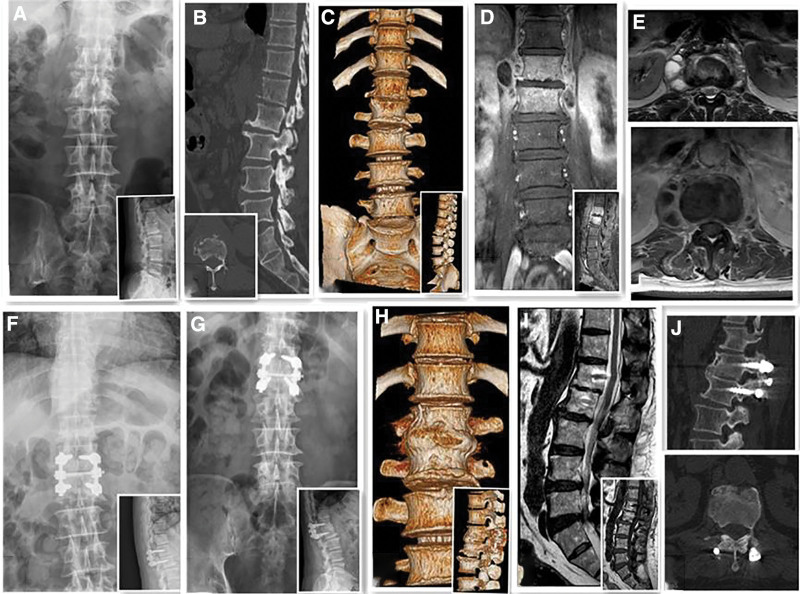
A 64-yr-old man, was admitted to the hospital mainly because of “low back pain with decreased muscle strength in both lower limbs” for 2 mo. Preoperative diagnosis revealed L1–2 brucellosis spondylitis, thus he was subjected to L1–2 simple bone graft internal fixation. (A) Preoperative X-ray films; (B and C) preoperative CT plain scan + reconstruction; (D and E) preoperative MRI; (F) X-ray films taken 3 mo after surgery; (G) lateral and frontal radiographs at last follow-up; (H and J) CT + reconstruction image at last follow-up; (I) MRI image at last follow-up.
